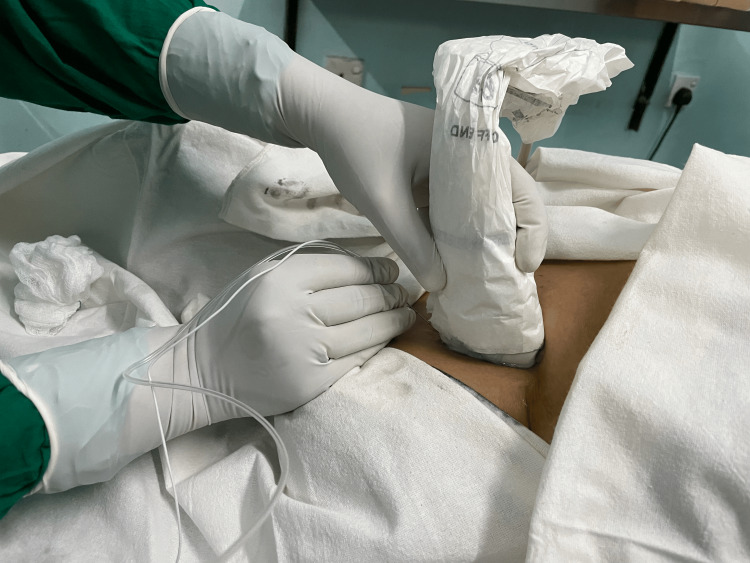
Ultrasound-guided PECS I/II block being administered PECS: pectoral nerve block
